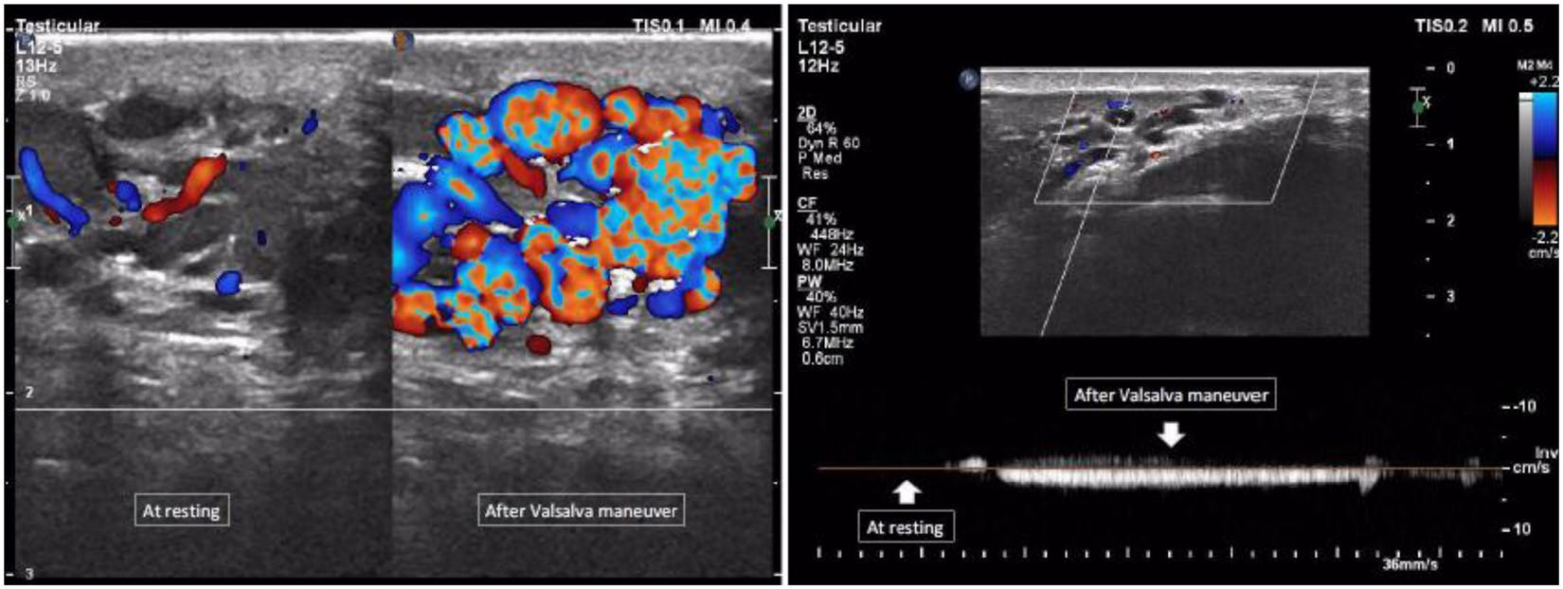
Augmentation pattern reflux: No color seen during rest, but the color was seen (red or blue) during the Valsalva maneuver, and in Doppler spectrum, venous flow was seen only during the Valsalva maneuver.
